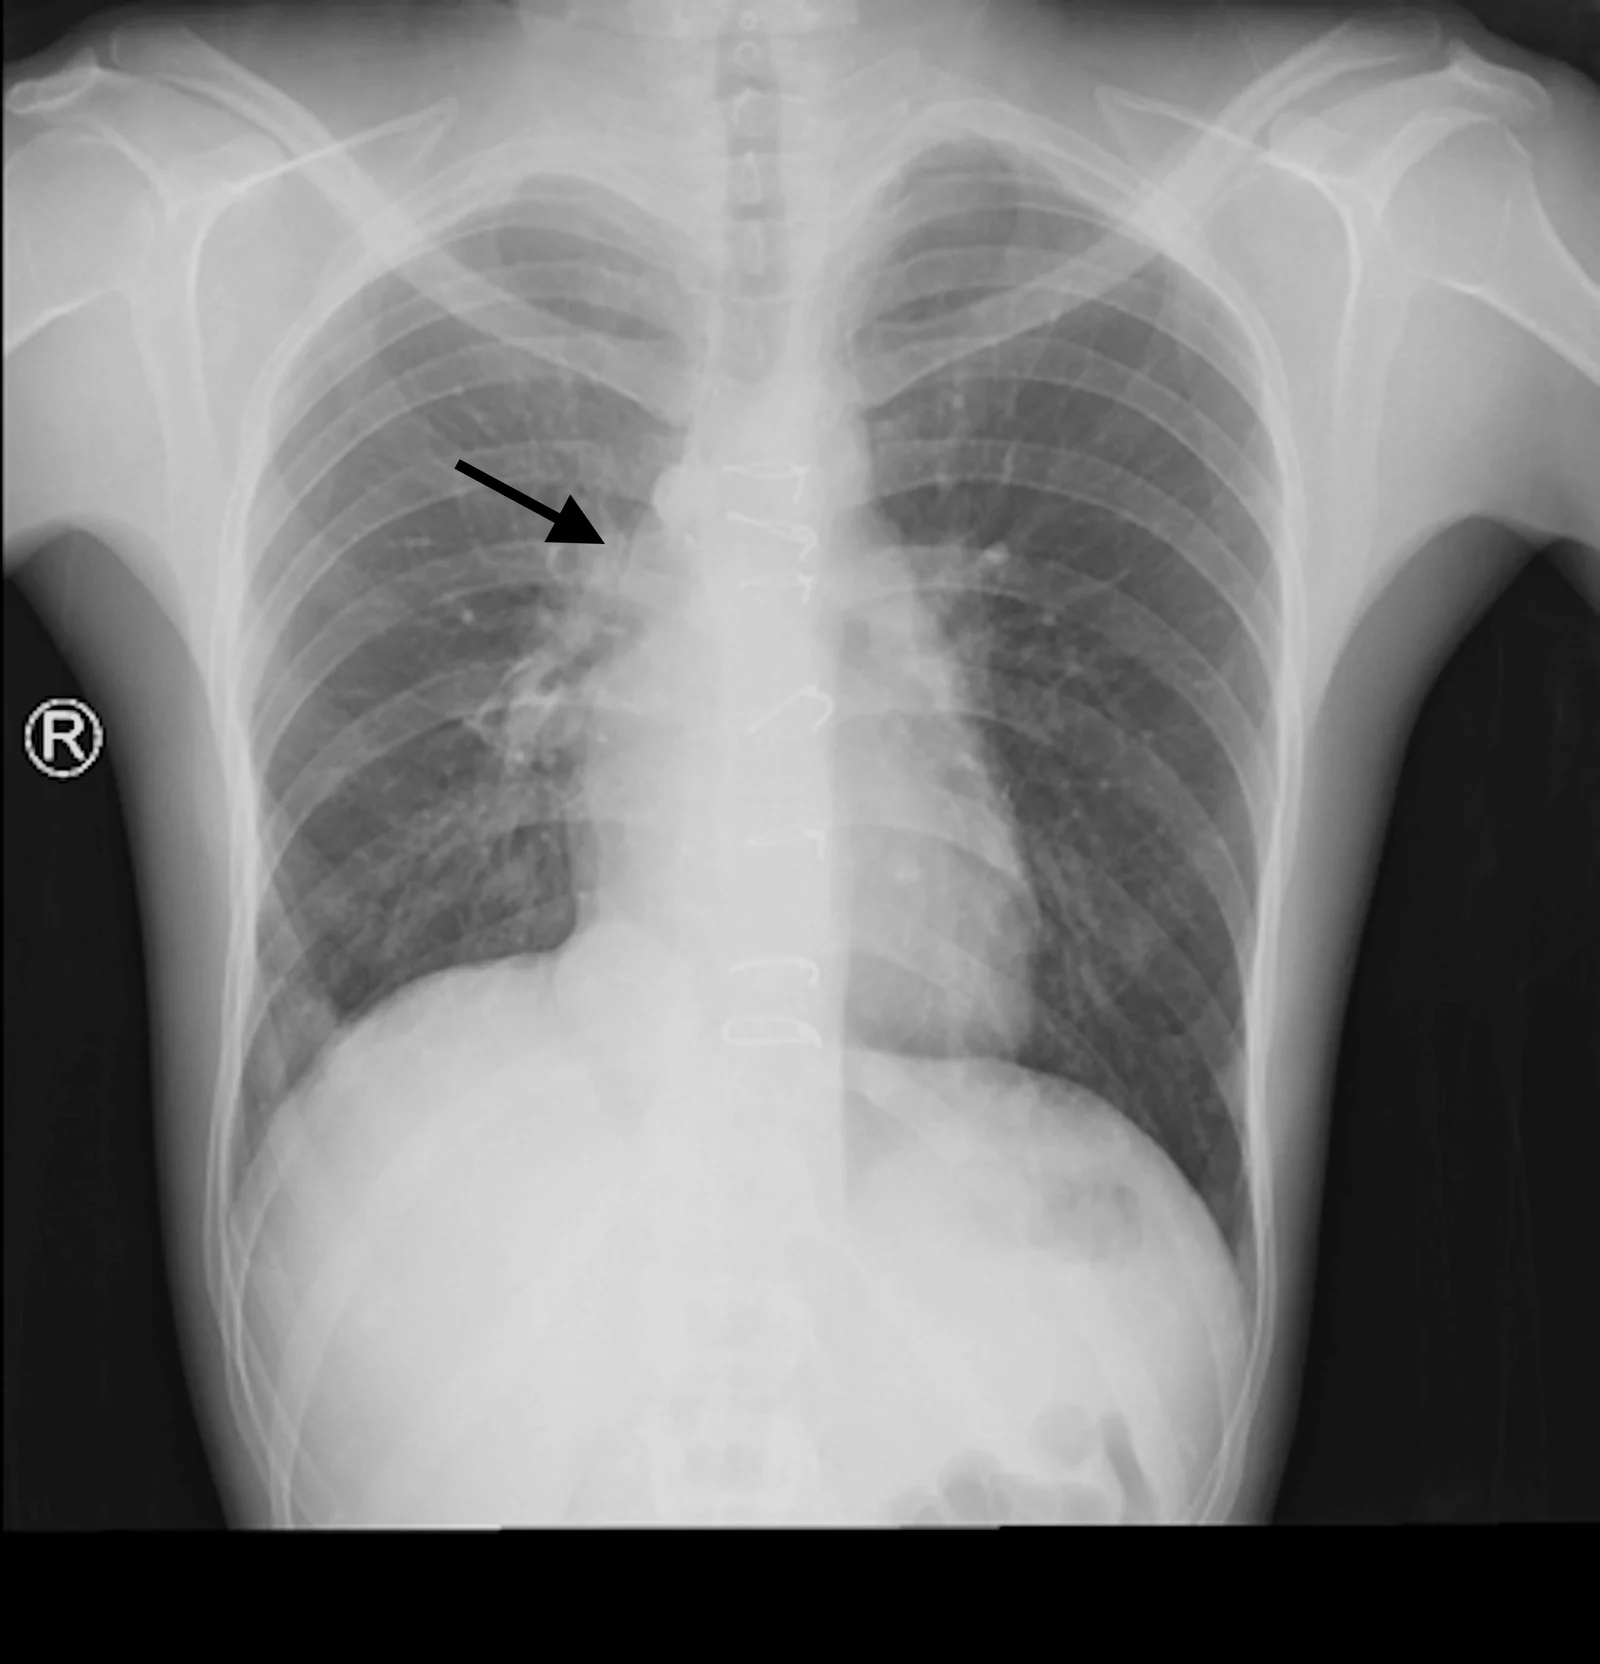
Postoperative chest x-ray showing clear lung fields and absence of the previously seen mediastinal mass. The arrow indicates the postoperative region previously occupied by the mass
